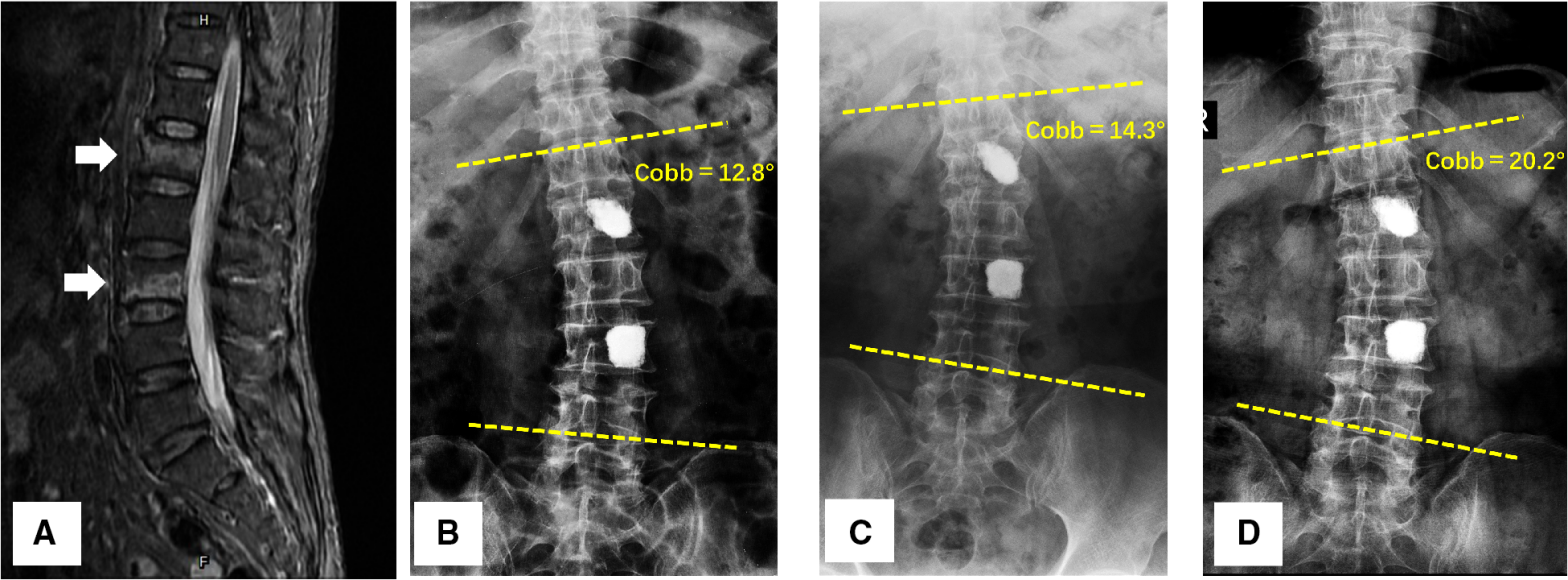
A 58-year-old female was diagnosed with L1 and L3 vertebral compressed fractures treated with unilateral PVP. (A) Preoperative T2-weighted MRI scan showed a higher-intensity signal within the fractured vertebral body; (B–D) 24 h after surgery, 6-month follow-up, and 48-month follow-up anteroposterior *x*-rays of lumbar spine, a progressive Coronal Segmental Cobb angle was observed with time.
